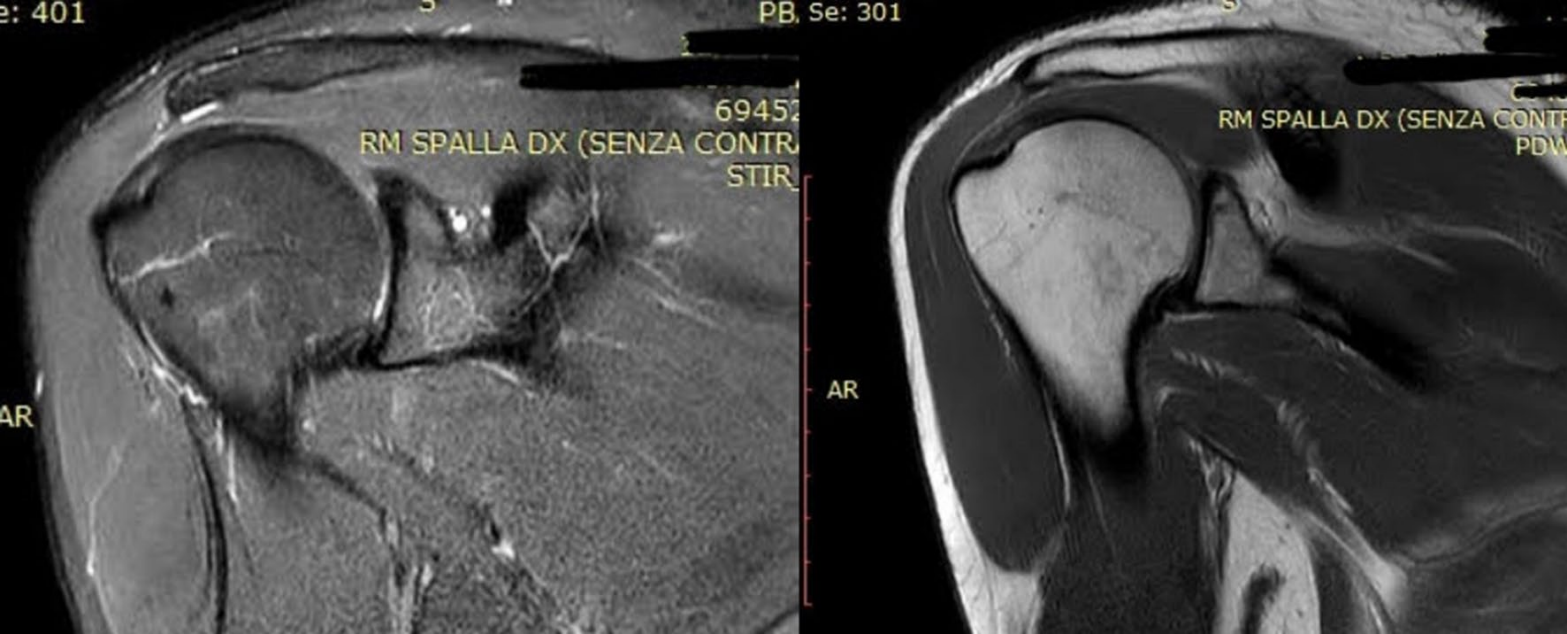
MRI Coronal STIR-weighted image and PD-weighted image of the supraspinatus tendons of an enrolled patient at T0
